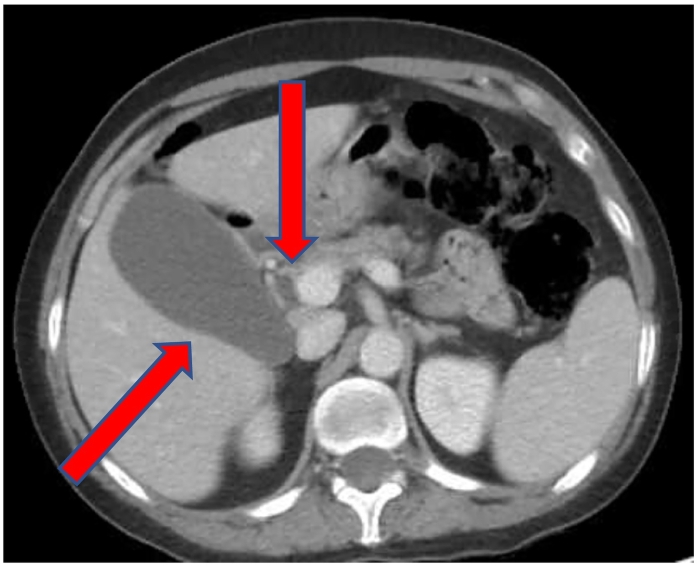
CT scan: Enlarged gallbladder overlying duodenal lipoma.
